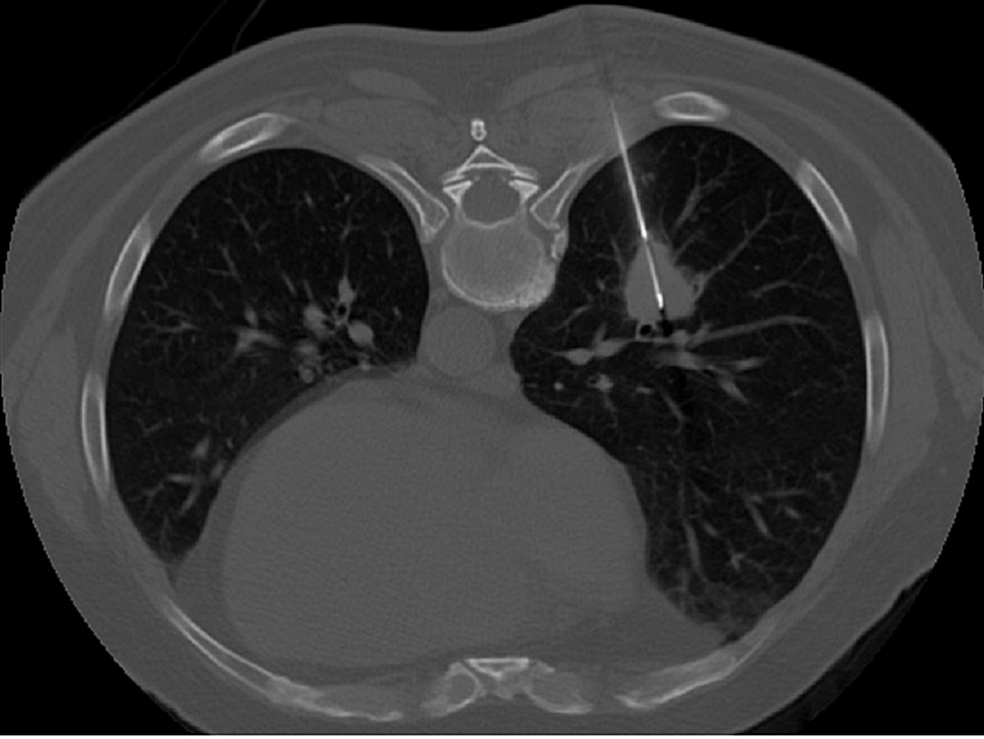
CT-guided lung biopsy of a right lung lesion (prone position). CT - computed tomography
